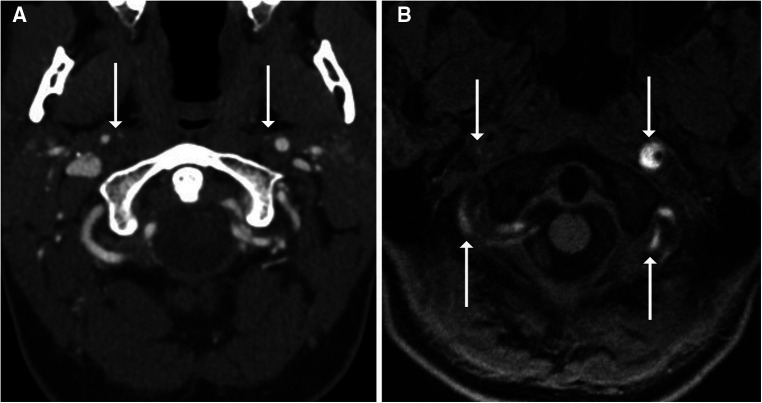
Imaging of a 28-year-old woman who presented with neck pain and headache immediately after delivering her first child. CTA (A) showed narrowing of the right more than left cervical internal carotid arteries with suspected crescentic intramural hematomas (arrows). MRA was performed with MPRAGE (B), which confirmed bilateral internal carotid artery and vertebral artery intramural hematomas, with many areas showing crescentic T1 hyperintense signal (arrows).
